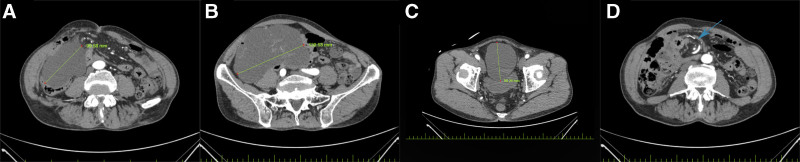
A selection of abdominal CTA images. Abdominal CTA revealed a large cystic mass in the right mid and lower abdomen, with mild enhancement of the cyst wall and septa (A–C). The arrow in the image indicates a localized mesenteric volvulus in the right mid-abdomen (D). CTA = computed tomography angiography.
